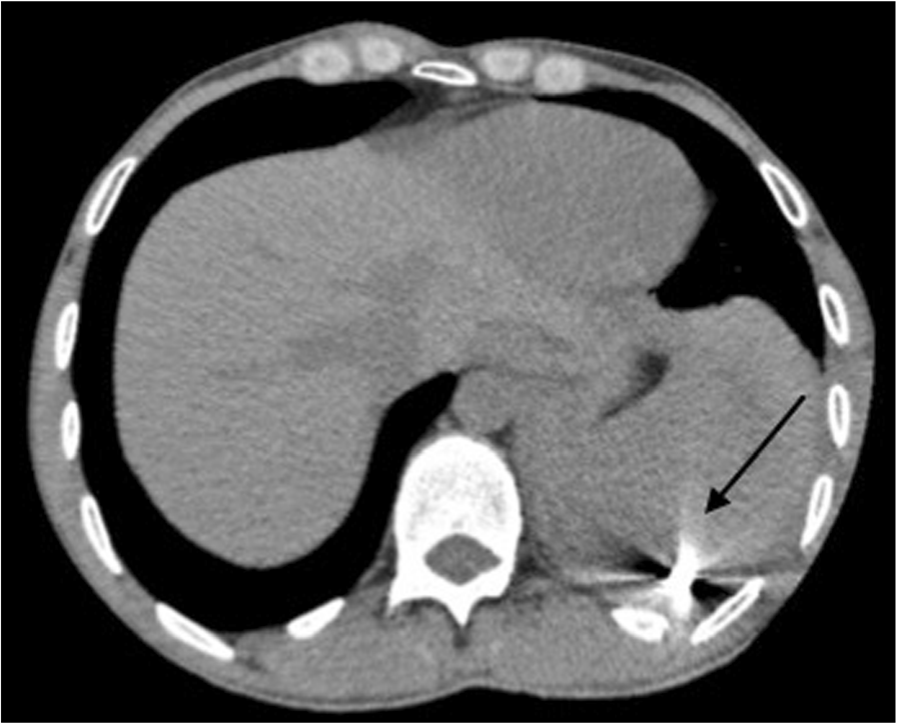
Chest computed tomography scan: evidence of a metal foreign body in the left chest cavity above the ipsilateral hemidiaphragm (the arrow indicates the metal foreign body)
